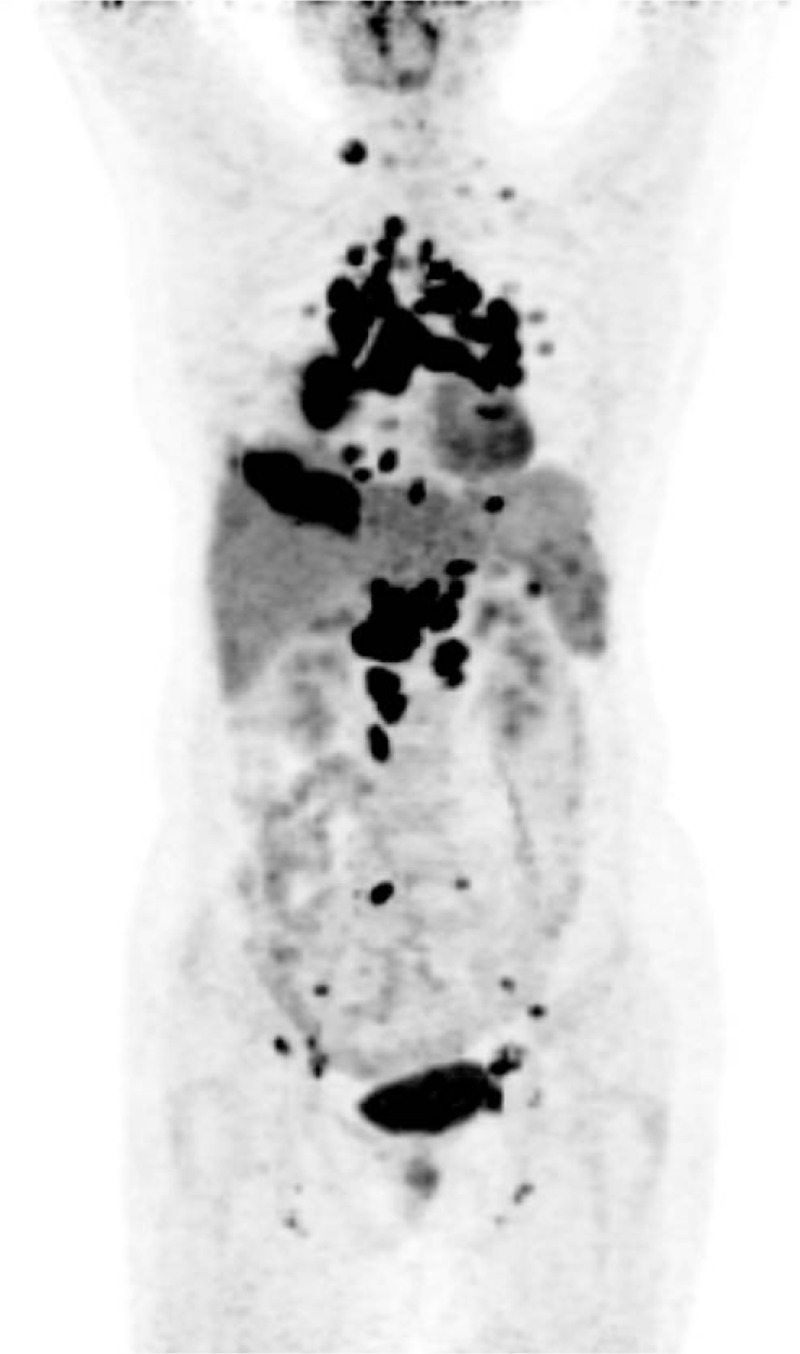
Maximum intensity projection image for F-18 FDG PET/CT showing intense accumulation of F-18 FDG in multiple lymph nodes, lung, liver, and spleen. F-18 FDG PET/CT = ^18^F-fluorodeoxyglucose positron emission tomography/computed tomography, SUV_max_ = maximum standardized uptake.
